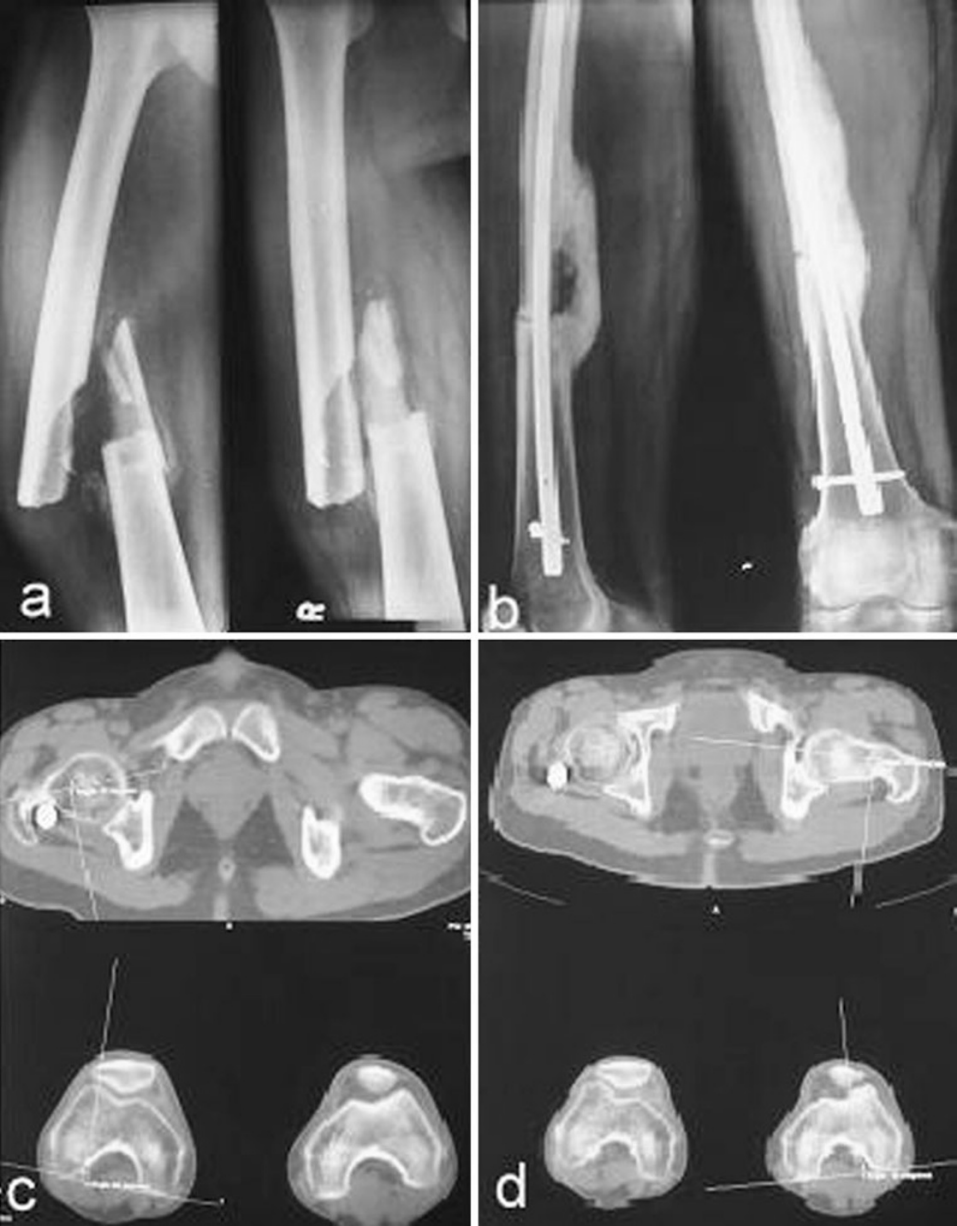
a Preoperative anteroposterior and lateral radiographs of thigh showing AO type B3 fracture and type III fracture according to Winquist–Hansen classification. b Postoperative anteroposterior and lateral radiographs showing union. c and d CT scan showed acceptable rotational alignment achieved in the patient (internal rotation deformity of 5°)
